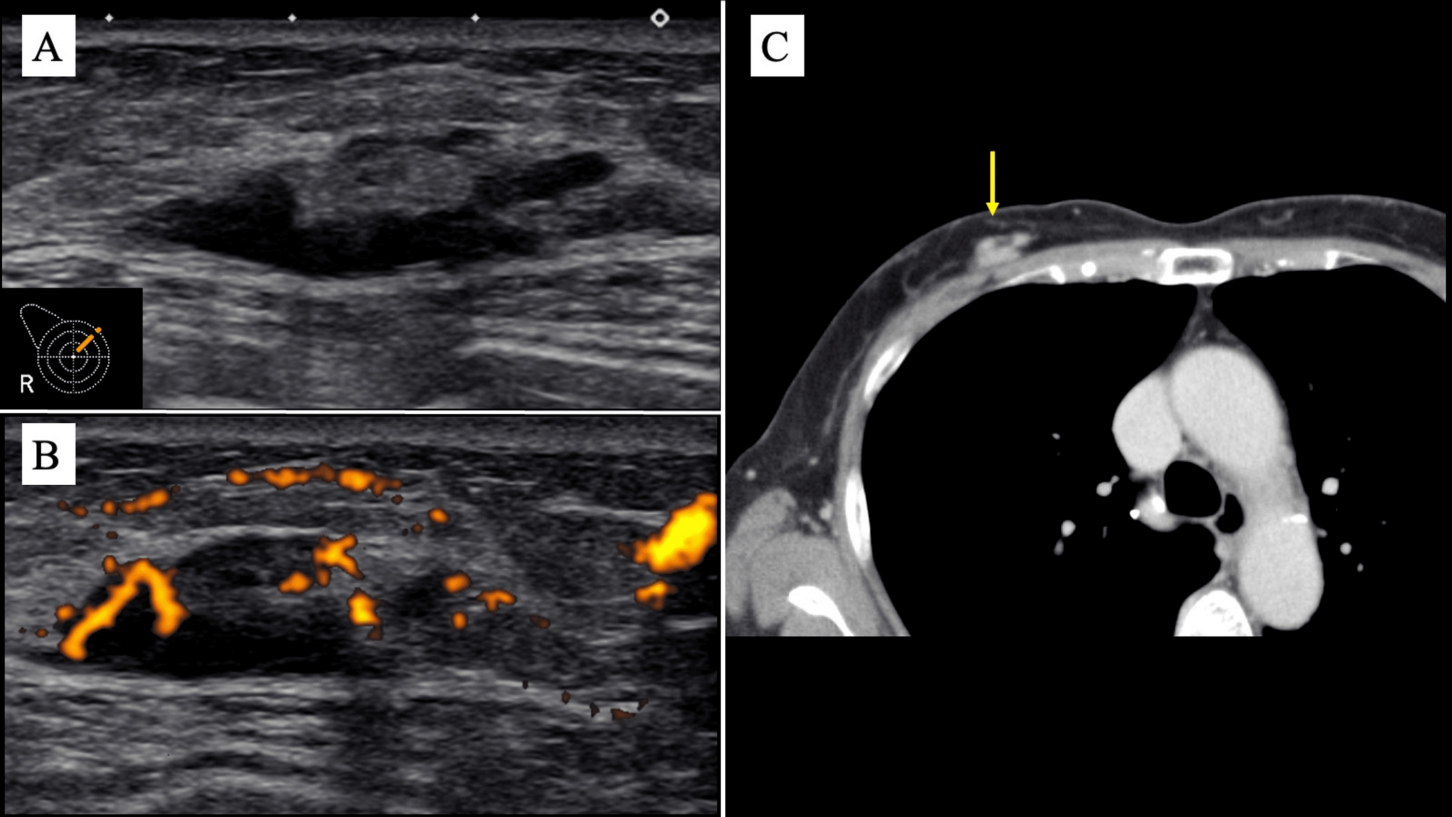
Ultrasonography (US) and contrast-enhanced computed tomography (CT) findings (A) The US demonstrated a mass with relatively uniform and extremely low echogenicity, in contrast to the typical ultrasound image of lobular carcinoma, which shows an irregular, hypoechoic mass. A septal structure was detected within the tumor, and (B) blood flow signals were abundant. (C) CT scans showed a lobulated mass composed of contiguous oval and irregular components, representing a homogeneously enhancing lobulated mass (yellow arrows). These findings are more indicative of malignant lymphoma than of invasive lobular carcinoma (ILC).
